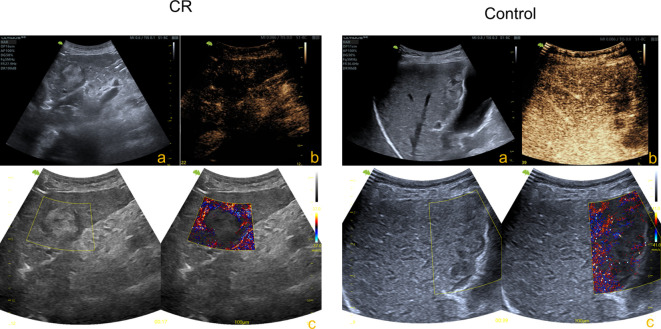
ULM examination in two groups showed different microvascular. (A) 2D ultrasound liver examination, localized lesions. (B) CEUS of lesions. (C) ULM of lesions.
